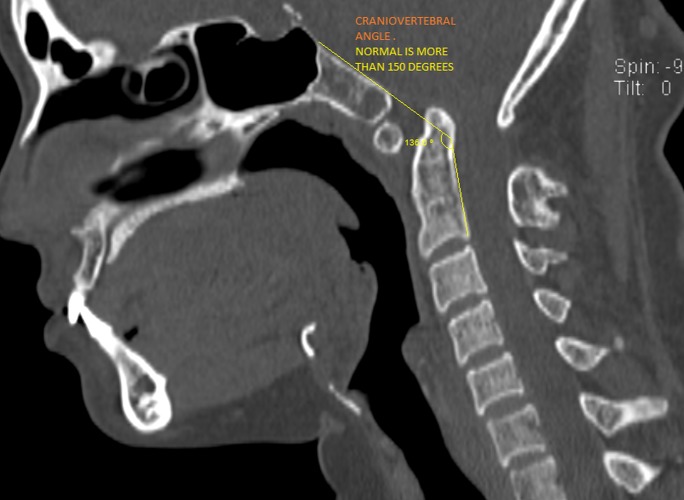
Sagittal computed tomogram showing reduced cranio-vertebral angle
